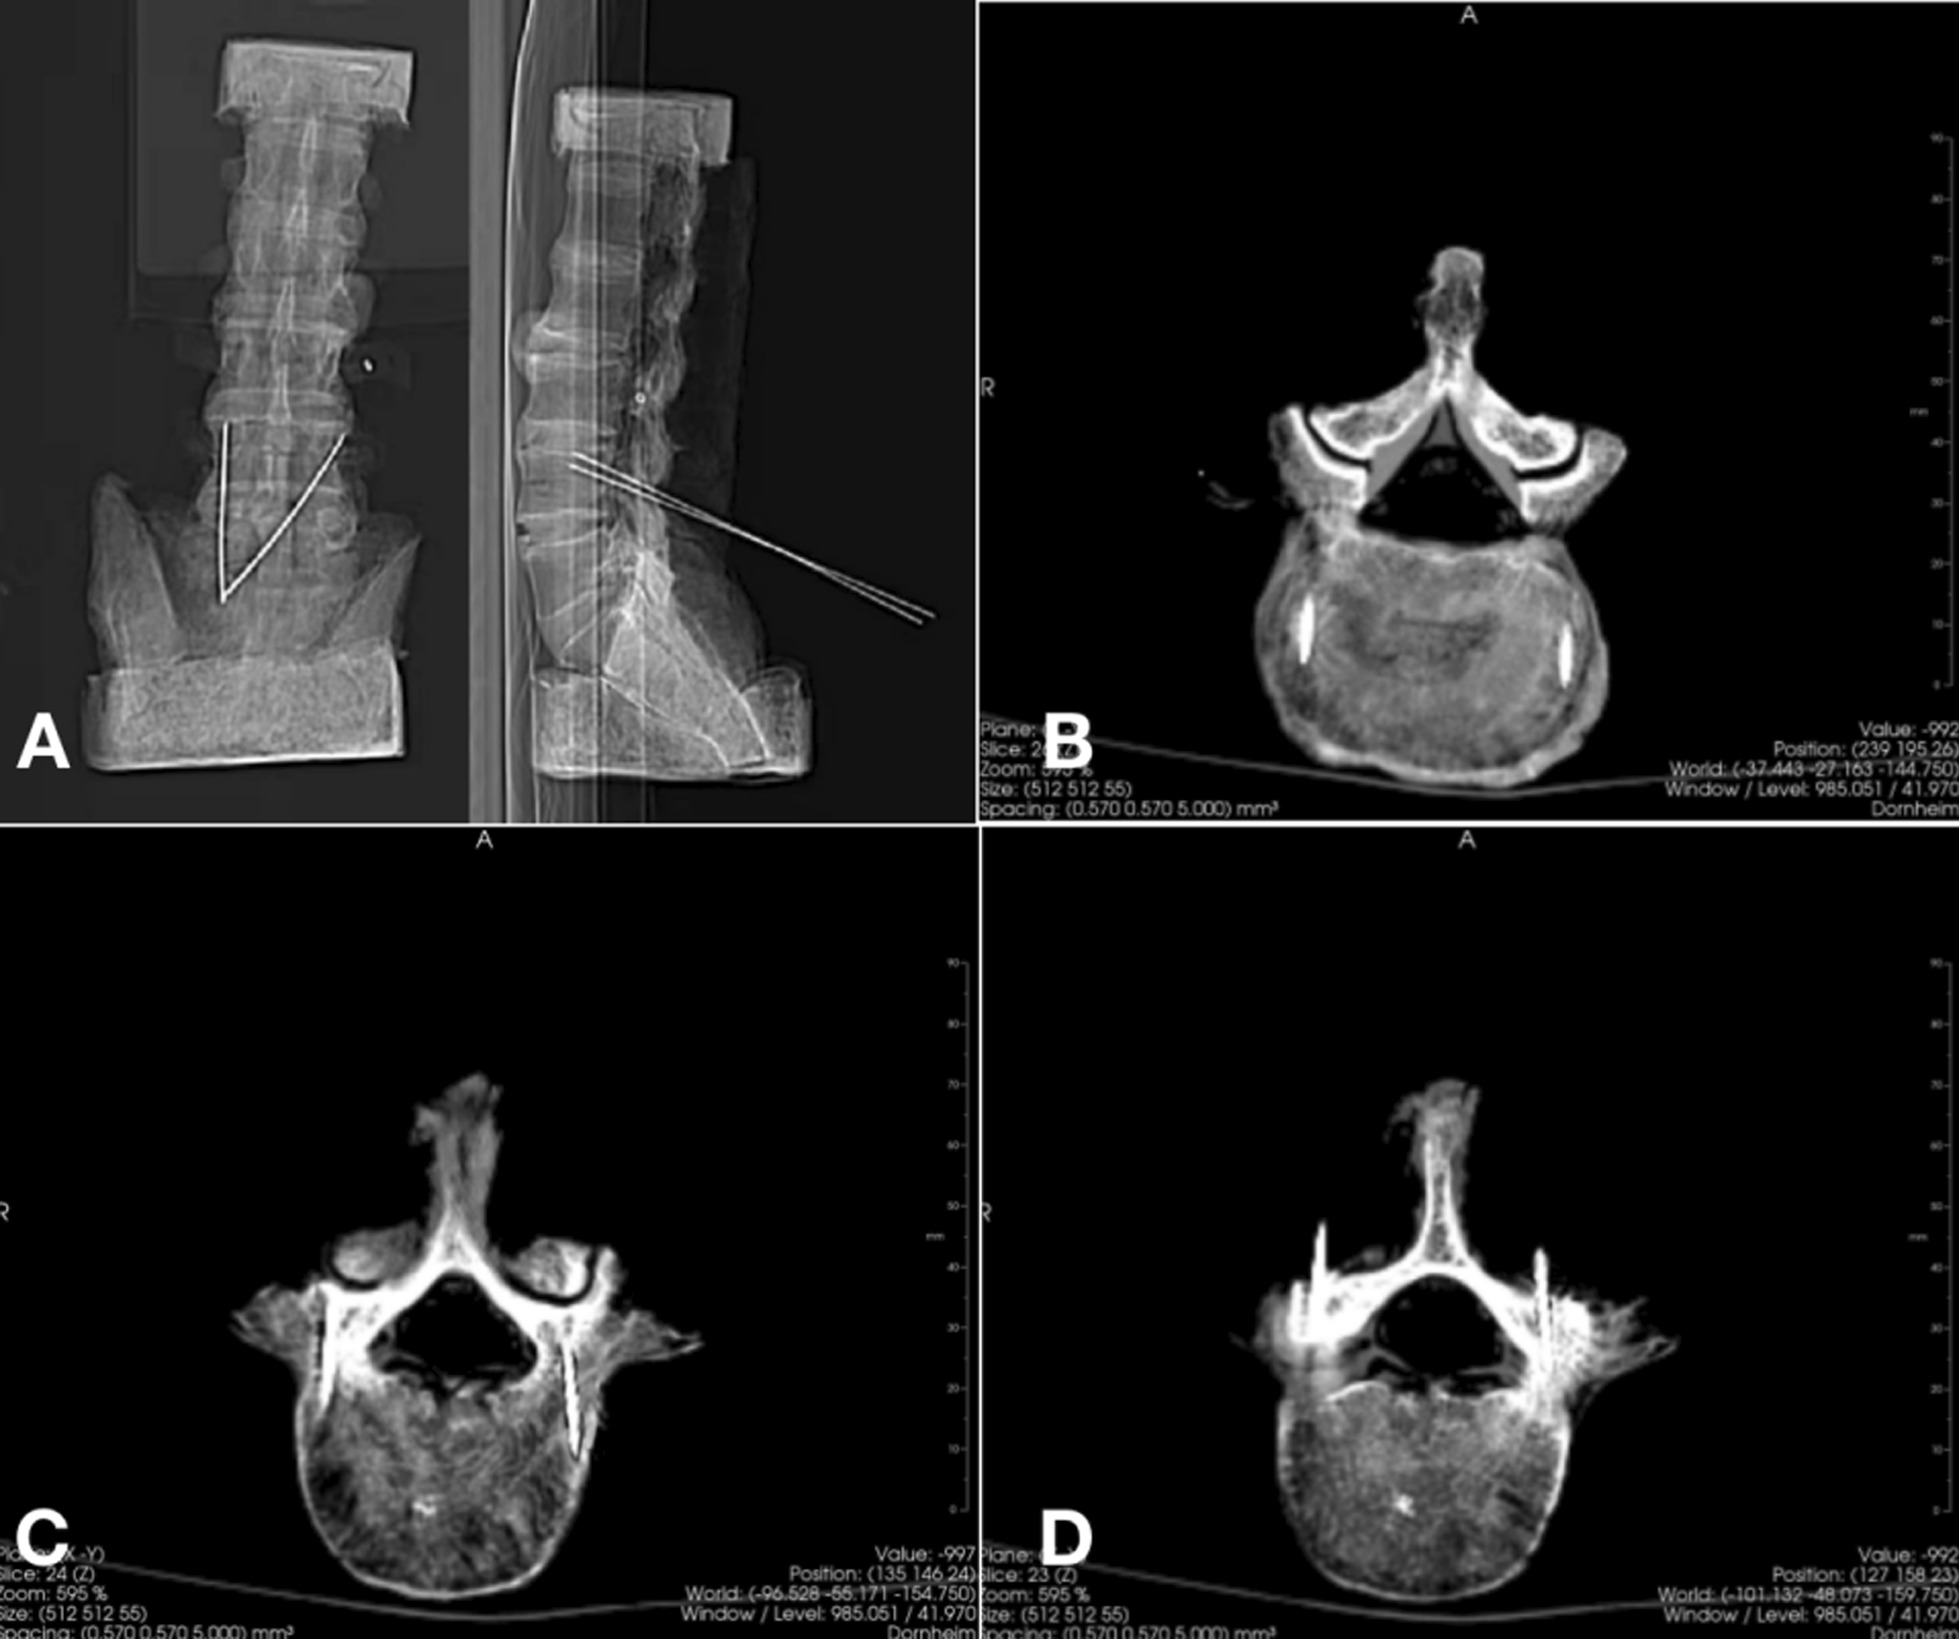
A CT scan location image; B the first layer of 1.5 mm K-wires in L4 vertebrae; C the second layer of 1.5 mm K-wires in L4 vertebrae and pedicle; D the third layer of 1.5 mm K-wires in L4 pedicle
